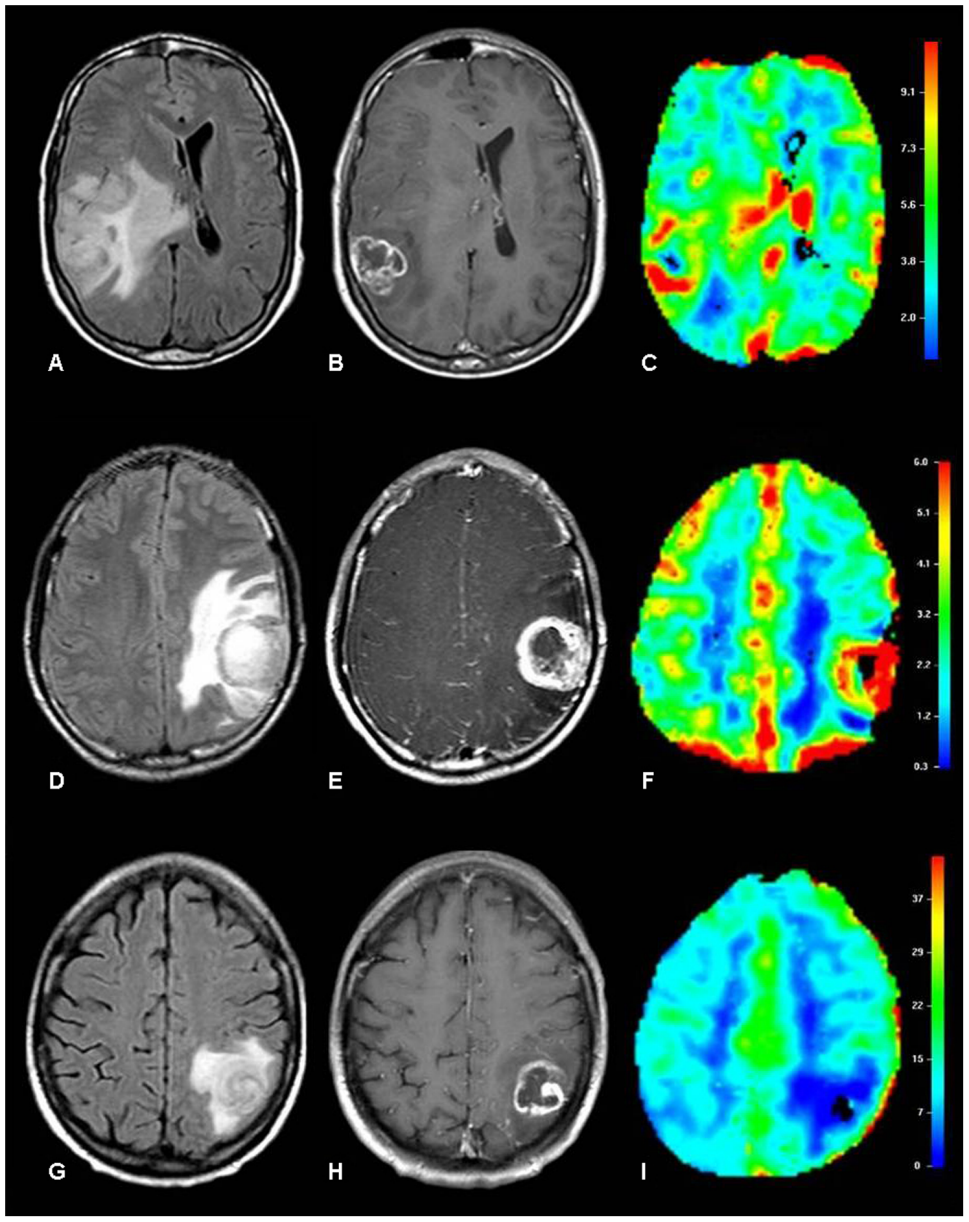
Examples of rCBV maps of neoplastic and infectious lesions. Glioblastoma multiforme in a 49-year-old man (A,B,C). Axial FLAIR image shows a mass lesion in the right parietal lobe surrounded by infiltrating and vasogenic edema (A), with irregular peripheral enhancement on axial contrast-enhanced T1-W image (B) and increased vascularity on the color-coded rCBV map (C). Metastatic melanoma in an 18-year-old man (D,E,F). Axial FLAIR image depicts a mass lesion in the left parietal lobe with perilesional vasogenic edema (D), irregular peripheral rim enhancement on the axial post-contrast T1-W image (E) and increased vascularity on the color-coded rCBV map (F). Cerebral toxoplasmosis in a 54-year-old woman (G,H,I). Axial FLAIR image shows a mass lesion in the left parietal lobe with surrounding vasogenic edema (G), irregular peripheral rim enhancement on the axial post-contrast T1-W image (H) and hypovascularity on the color-coded rCBV map (I).
